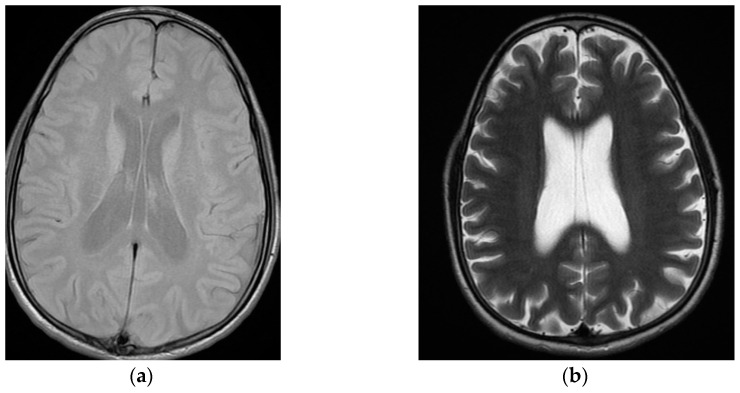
Sequential neuroimaging showing mild progression of diffuse cortical cerebral atrophy with cavum septi pellucidi, cavum vergae, and cavum veli interpositi: (a) first brain magnetic resonance imaging (T1), at the index psychiatric admission; (b) second brain magnetic resonance imaging (T2), at treatment-resistant schizophrenia outpatient appointment, ten years later.
